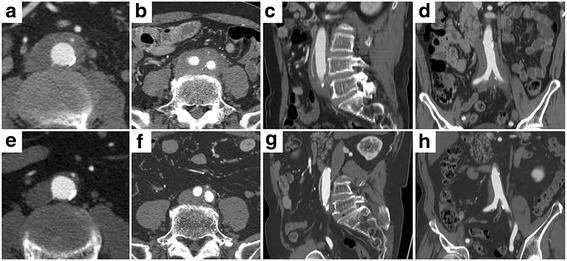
Contrast-enhanced CT (early phase) of IgG4-related perivascular lesions in an 80-year-old man. Walls of the abdominal artery (a) and common iliac arteries (b) showed thickening at diagnosis (a, b transverse plane; c sagittal plane; d coronal plane). Steroid therapy reduced abdominal wall thickness (e, f transverse plane; g sagittal plane; h coronal plane)
